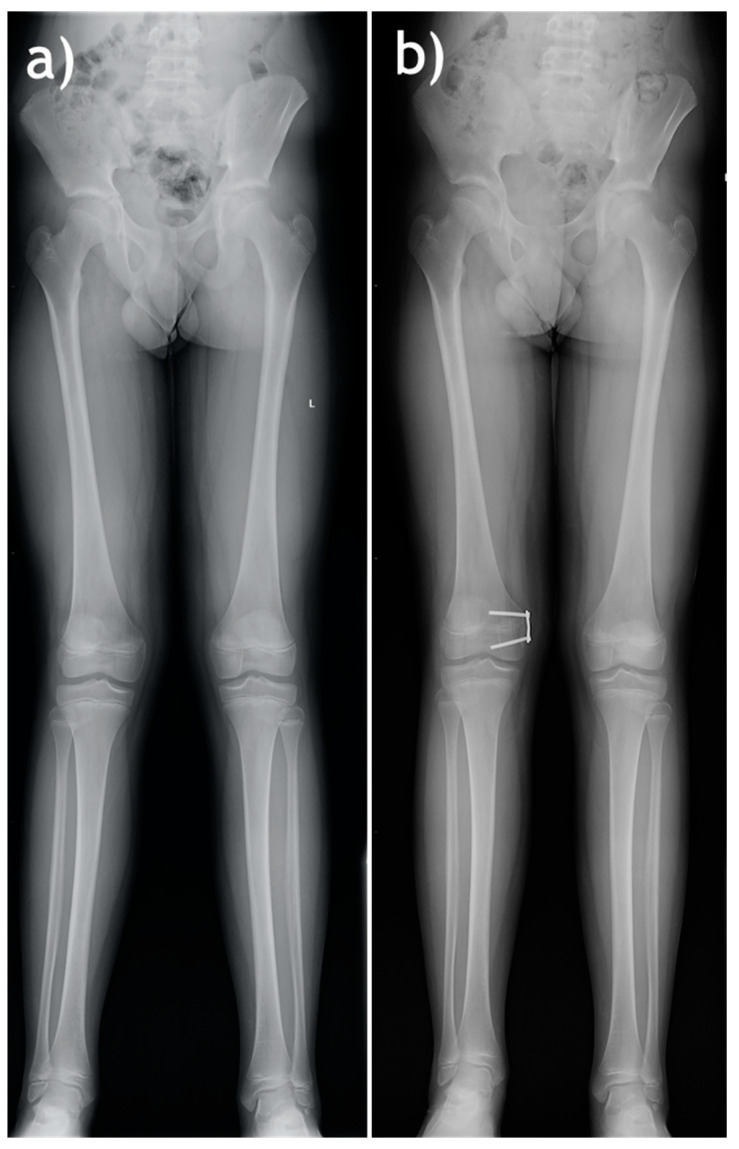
A sample patient with genu valgum—preoperative (a) and follow-up after hemiepiphysiodesis (prior to implant removal) X-ray images (b).
